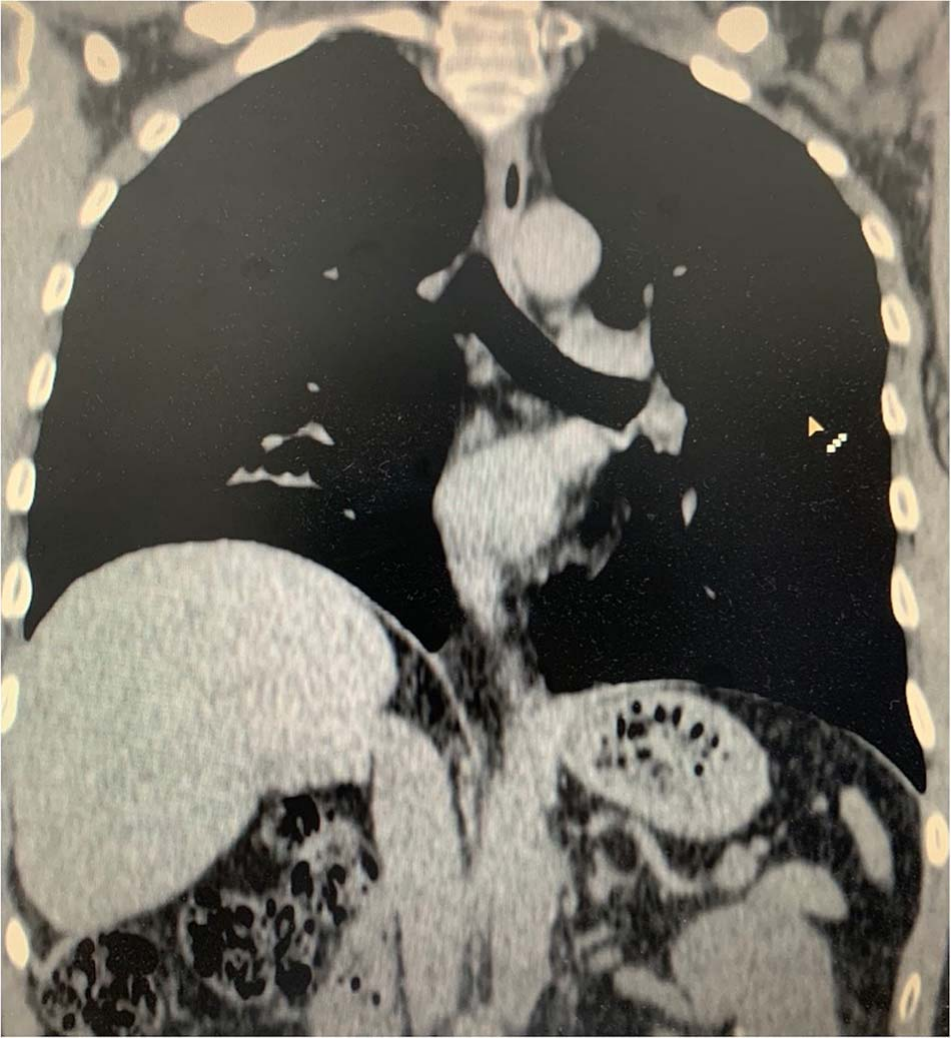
Chest CT image for re-evaluation after 5 months.
